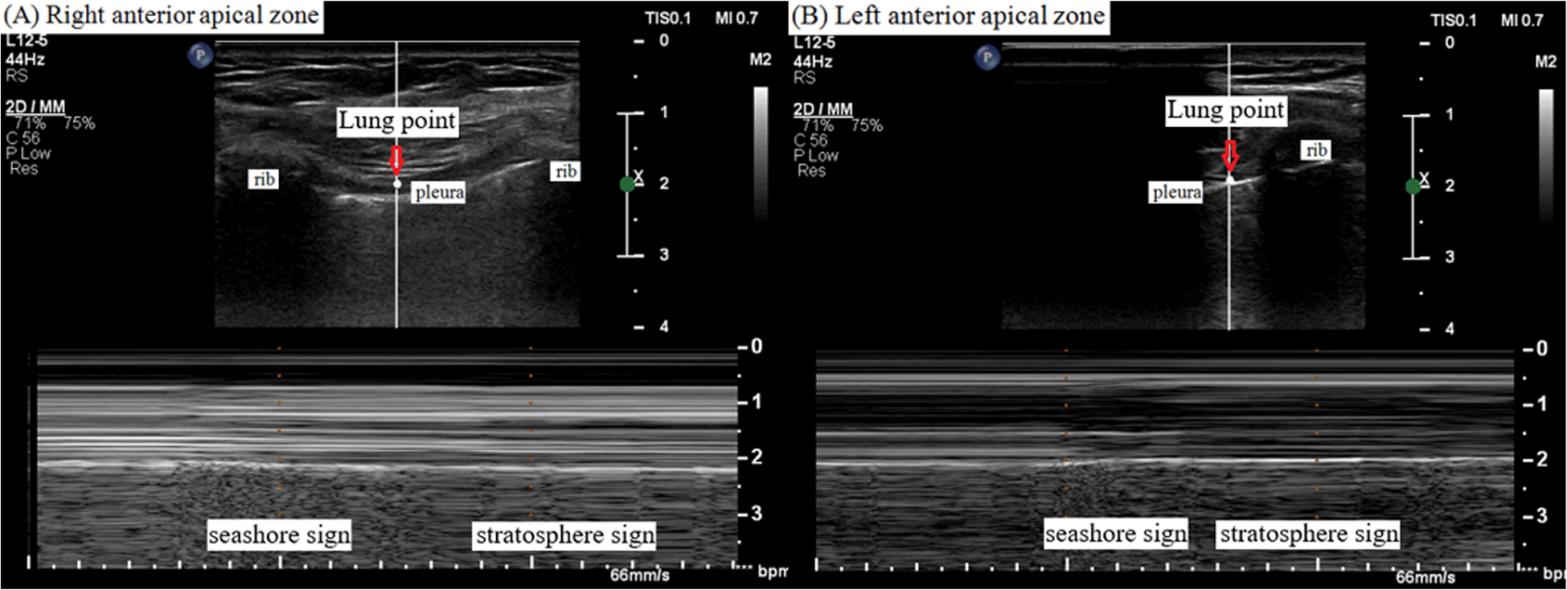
Lung point on point-of-care lung ultrasound. a Right anterior apical zone. b Left anterior apical zone. Arrows indicate the lung point where the normal lung pattern (i.e., seashore sign) replaces the pneumothorax pattern (i.e., stratosphere sign) with inspiration
